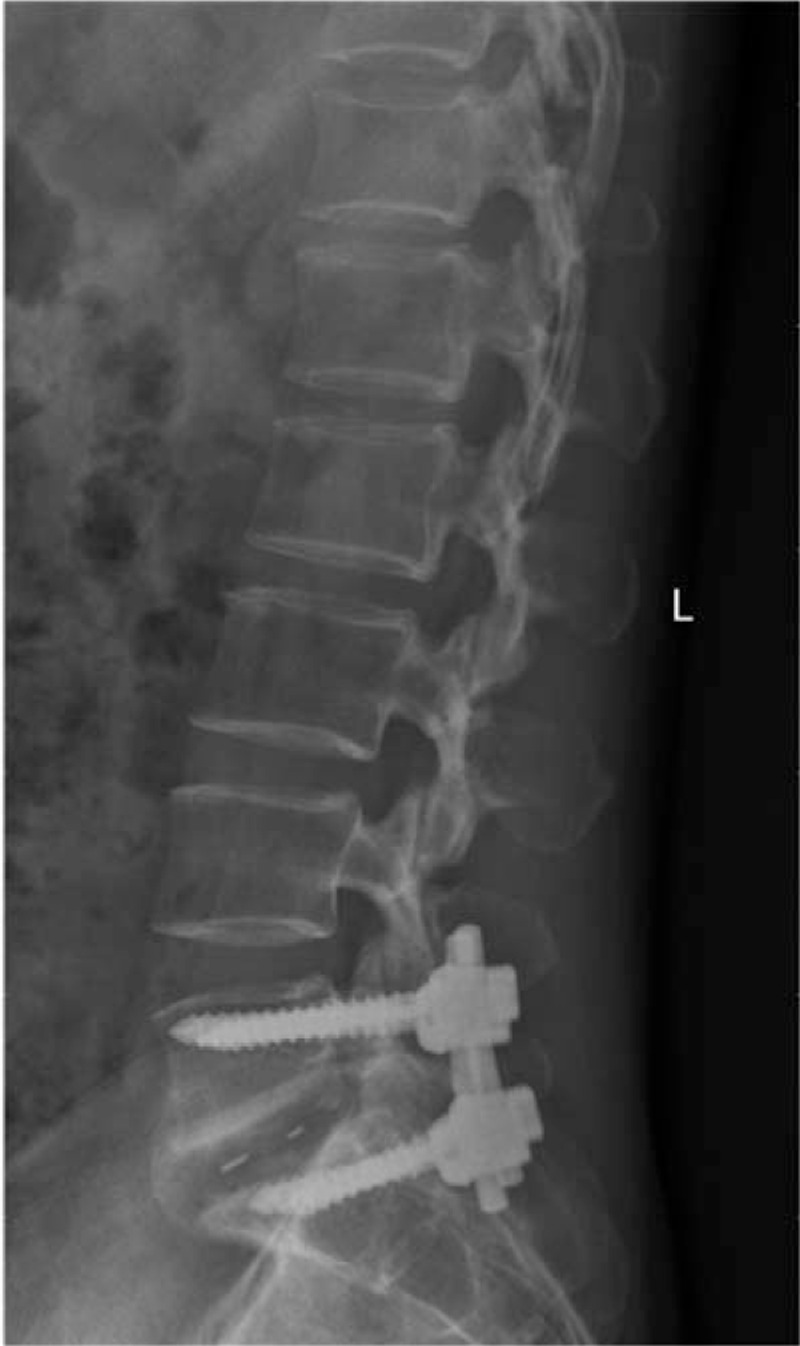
Lumbar vertebral X-ray: normal findings (arrow).
